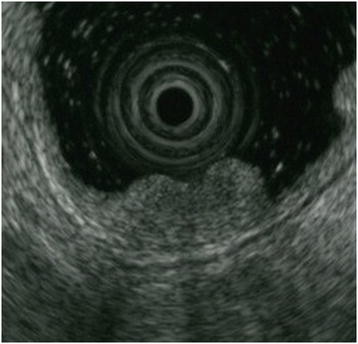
Endoscopic ultrasonography findings. Endoscopic ultrasonography (20mHz miniprobe) revealed that the duodenal wall was delineated into five layers. The tumor was visualized as a low echoic mass mainly involving the second or third layers but not the fourth or deeper layers.
